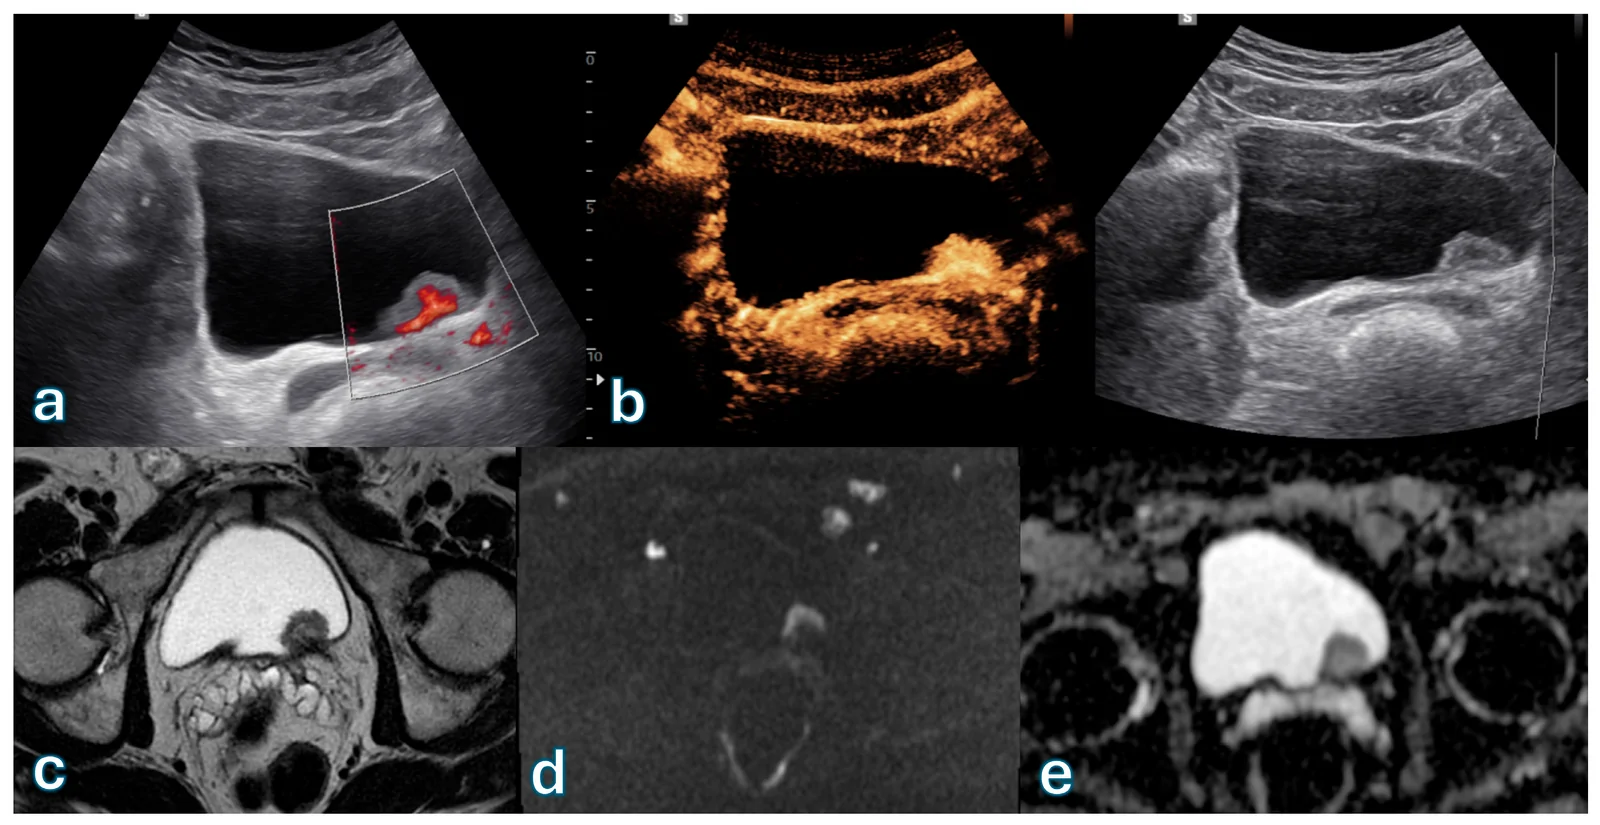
CEUS detection of the vascular stalk in a papillary bladder tumor. (a) Color Doppler ultrasonography demonstrates vascular flow at the base of the lesion. (b) Split-screen contrast-enhanced and gray-scale ultrasonography shows enhancement of a thin vascular pedicle extending from the bladder wall into the tumor, consistent with a positive CEUS vascular stalk sign. Corresponding multiparametric MRI includes axial T2-weighted imaging (c), diffusion-weighted imaging (d), and the apparent diffusion coefficient map (e). The lesion shows restricted diffusion, with high signal intensity on diffusion-weighted imaging and corresponding low signal intensity on the ADC map. The stalk is more conspicuous on real-time CEUS than on the corresponding MRI images.
